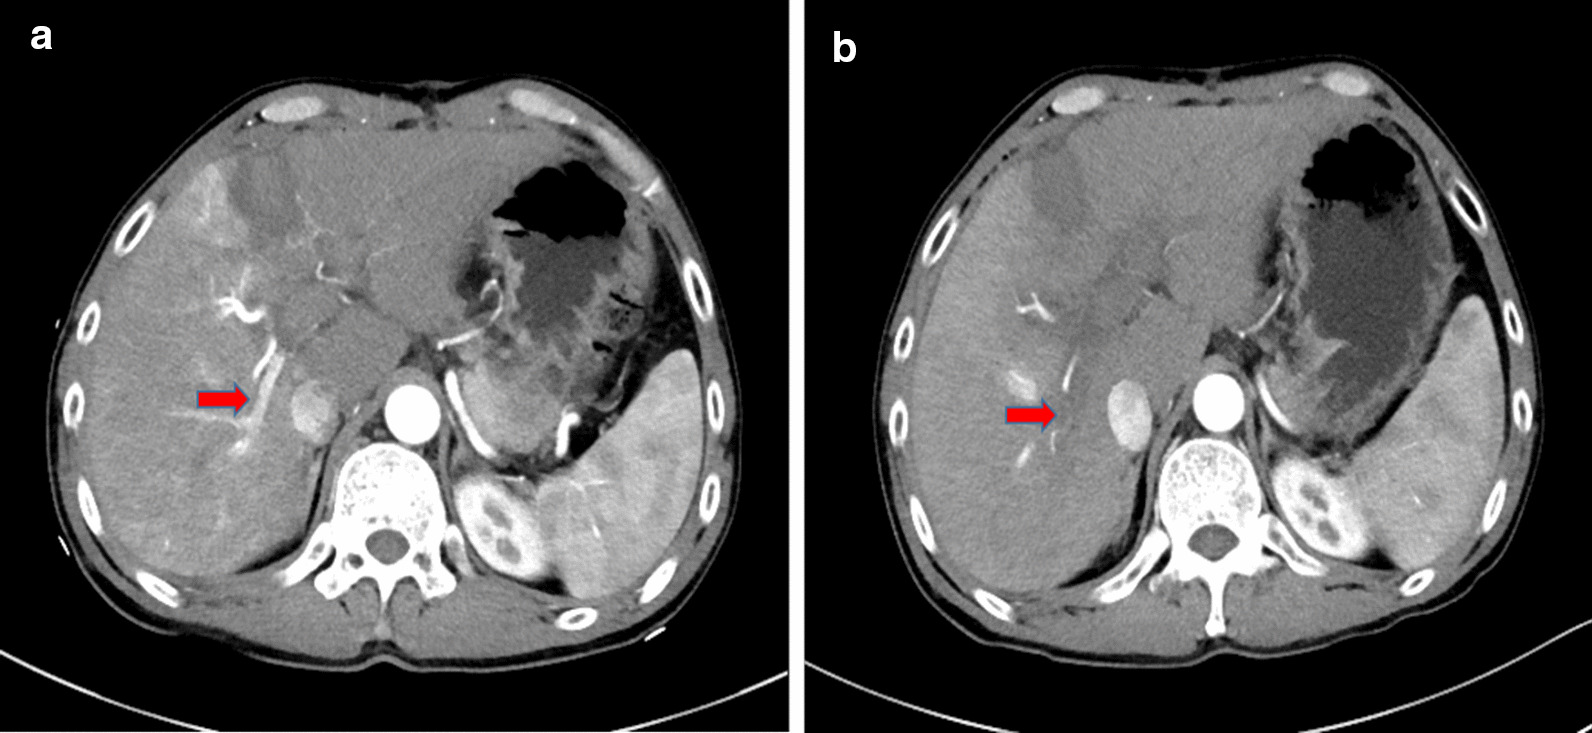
a First week after the RALPPS stage-1, ultrasound imaging showed an arterioportal shunt (APS) in the right ligated lobes [red arrow]. Note: RALPPS, radiofrequency-assisted ALPPS (associating liver partition and portal vein ligation for staged hepatectomy). b After three sessions of rescue percutaneous ethanol injection (PEI) with 10 mL ethanol each time, the APS disappeared [red arrow]
